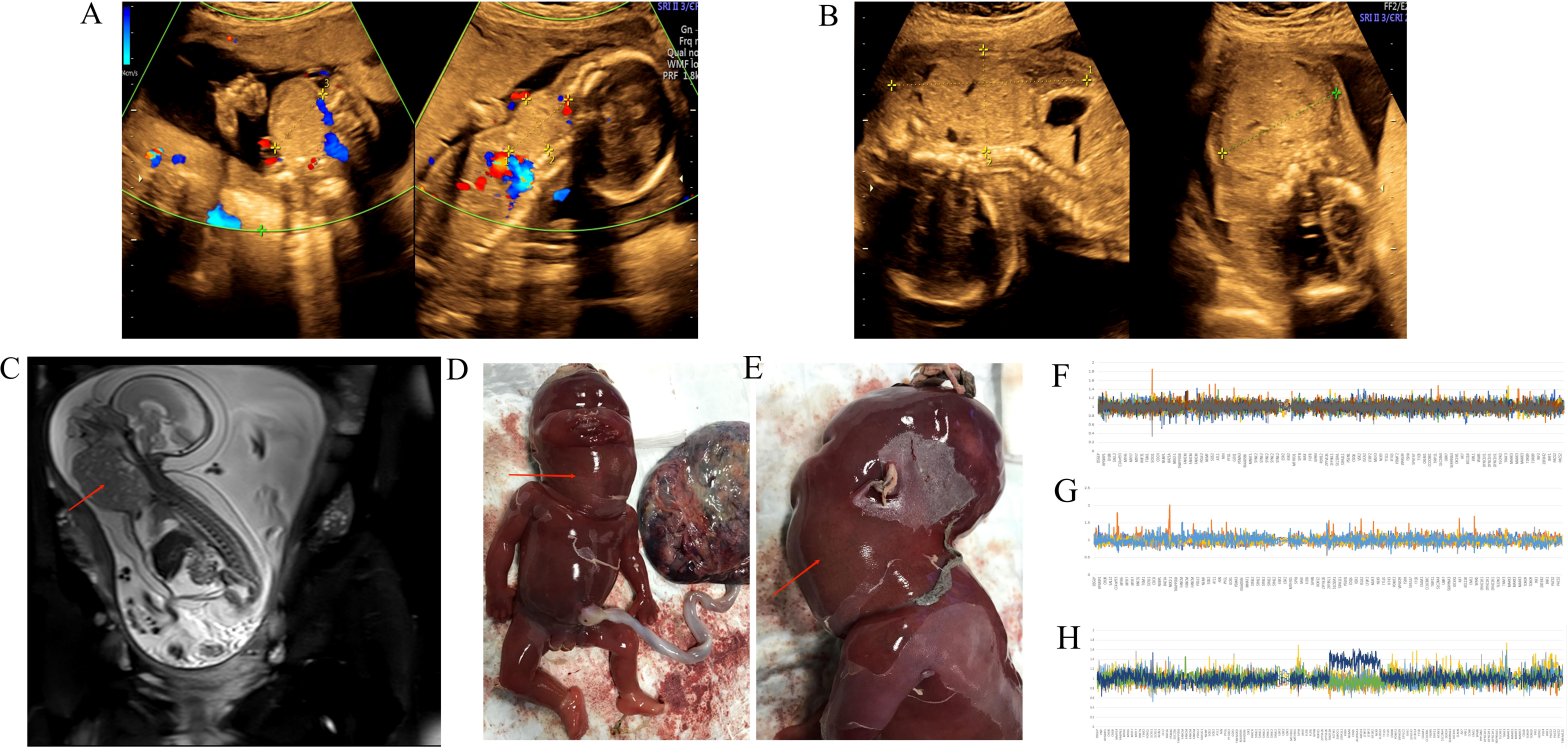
(A, B) 4D ultrasound scan showed the anterior solid neck mass of the fetus; (C) MRI of a nearly round heterogeneous tumor in front of the fetal neck with T2W image causing compression of the lung; (D, E) front and side views of the fetus with a prominent huge neck mass; (F–H) The map of copy number variation sequencing; (F) the father; (G) the mother; (H) the fetus in the first pregnancy.
